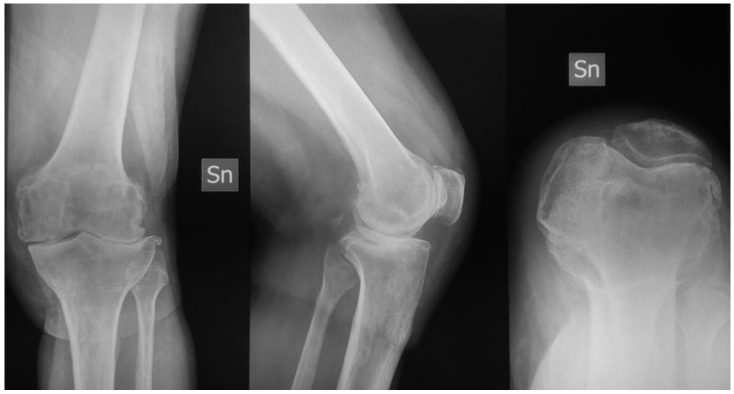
Preoperative X-rays of a 66 years-old woman undergoing TKR for knee osteoarthritis.
